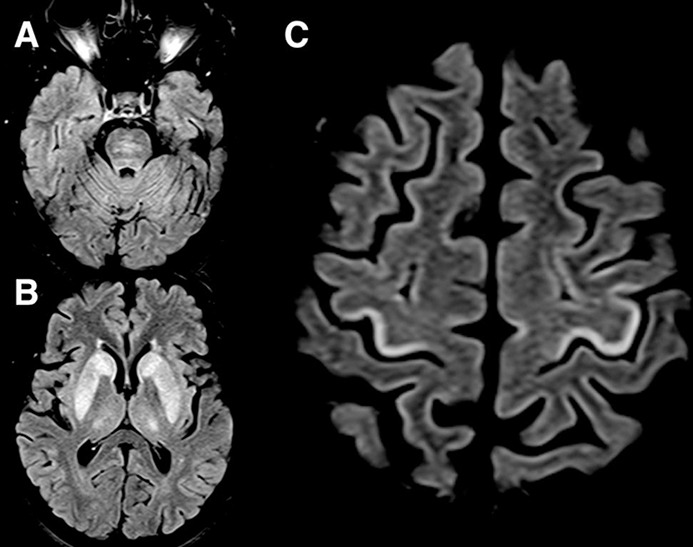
MR scan of the brain. Axial fluid-attenuated inversion recovery (FLAIR) images show symmetrical pontine (A), basal ganglia (B) and a ‘ribbon’ of cortical T2 hyperintensity in the posterior precentral gyri (C). The precentral gyral changes are strikingly similar to a focal pattern of MRI changes described in cases of amyotrophic lateral sclerosis.
